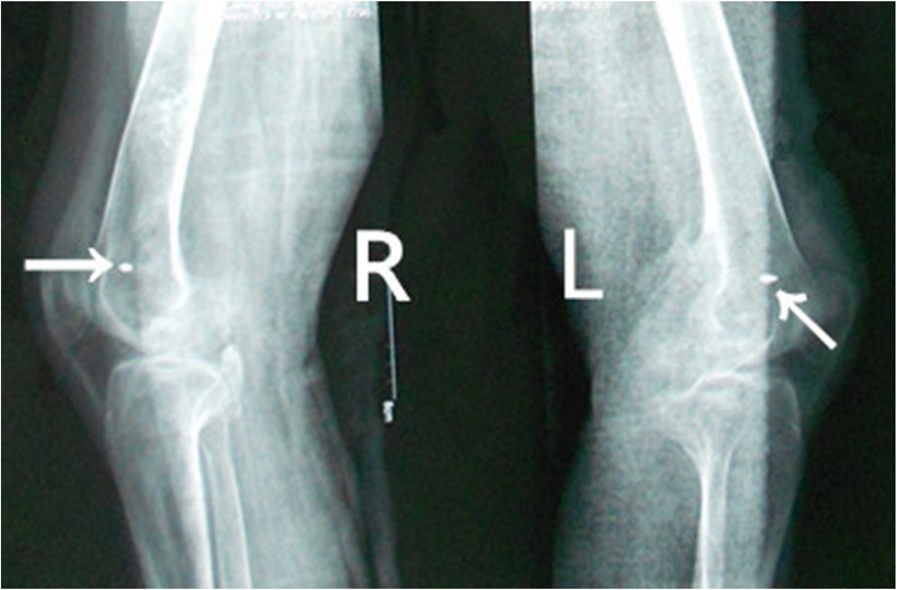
Postoperative radiographs of lateral radiographs of knees shows no loosening of rivets and no recurrence 13 moths after the excision of the ossific mass.
